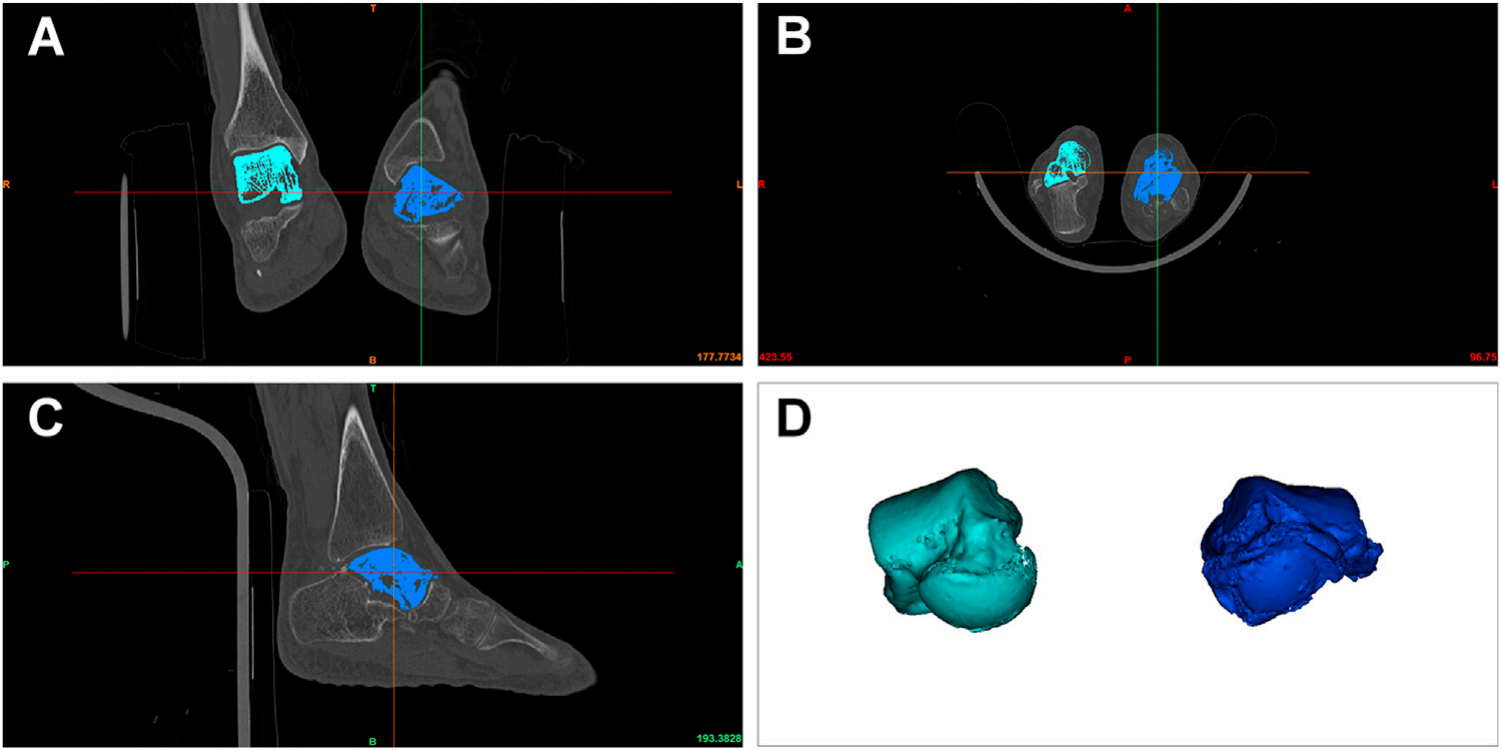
DICOM files obtained *via* CT were processed using Mimics software. Bilateral talars were located based on CT data (A–C), and three-dimensionally reconstructed (D).
